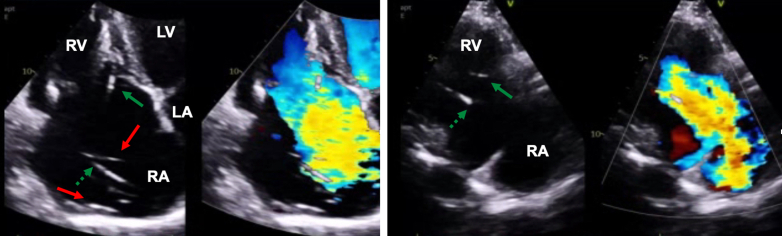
Transthoracic Echocardiographic Assessment of Tricuspid Regurgitation Apical 4-chamber view (left panel) and right-sided 2-chamber view (right panel) showing torrential tricuspid regurgitation with main central jet and annular and right ventricular dilatation. Notice the presence of 2 right atrial leads (red arrows) and 2 right ventricular leads with different course, one anteroseptal (solid green arrow) versus one posterior (dashed green arrow); see also Video 1. LA = left atrium; LV = left ventricle; RA = right atrium; RV = right ventricle.
